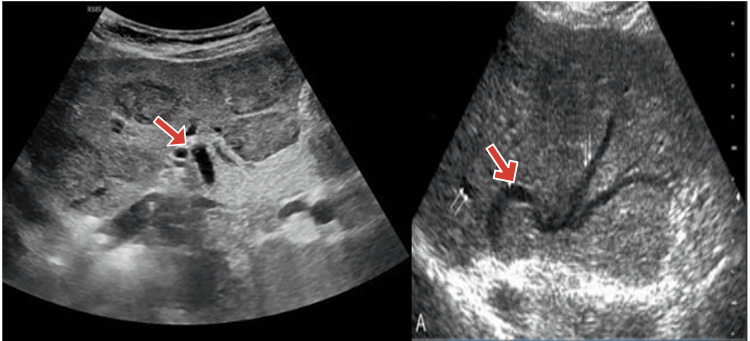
Ultrasound showing Liver With Dilated Biliary Ducts This part of the ultrasound shows a transverse section of the liver and biliary tree. The dilated bile ducts can be visualized, which is suggestive of biliary obstruction. The intrahepatic bile ducts are prominently shown by the red arrows.
